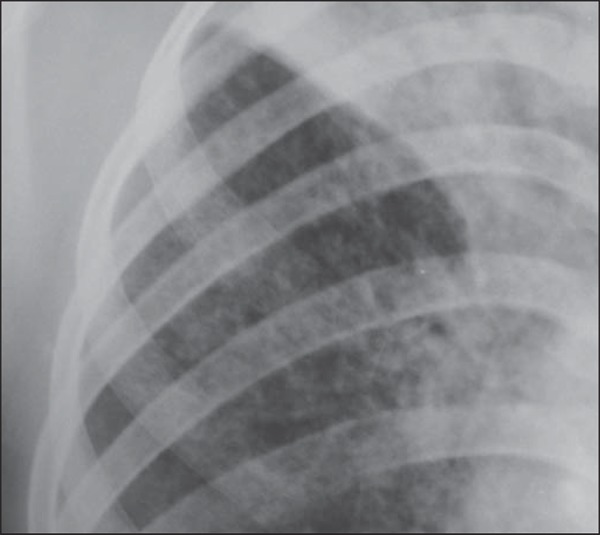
Chest radiography of a 14-year-old young girl showing the presence of pulmonary
